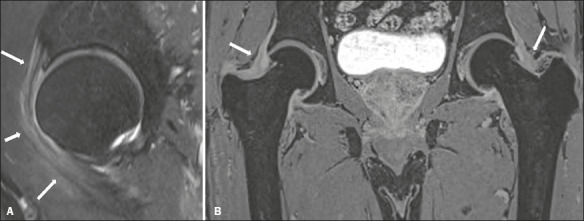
MRI of the right hip in a 58-year-old female with PMR. A: Sagittal T2wFS
